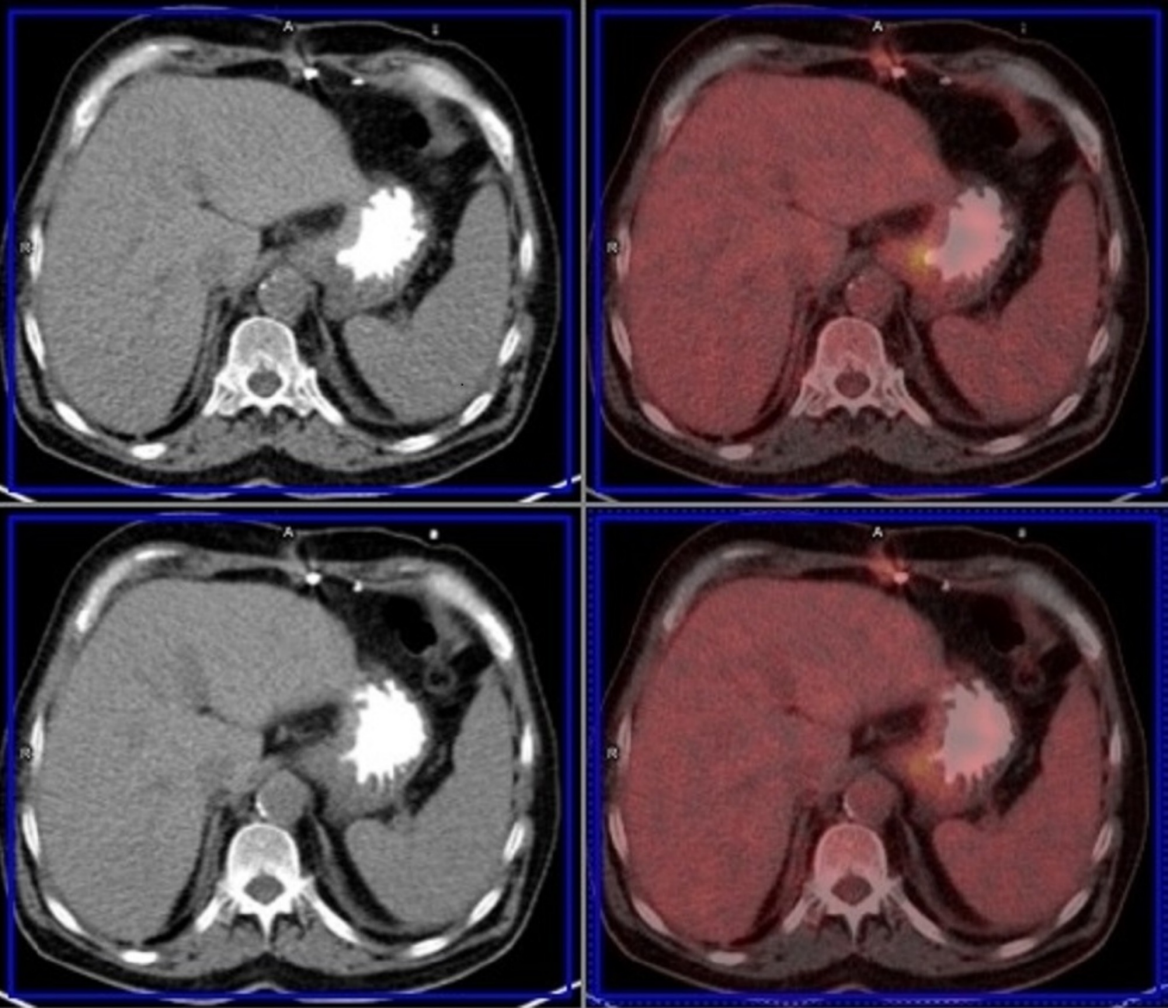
Fusion images of the gastric tumor Gastric tumor involving the posterior wall with elevated ^18^fluorodeoxyglucose metabolism in fusion images on positron emission tomography-computed tomography (PET-CT).
